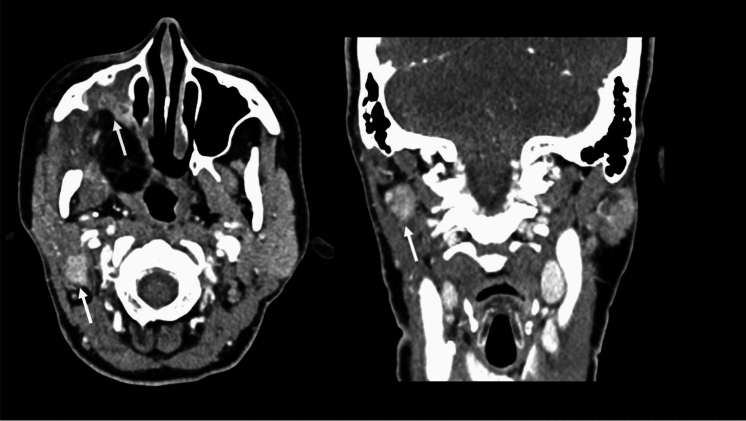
Axial and coronal views of post-operative CE-CT after 8 weeks in Patient with pT4aN3b superior right retromolar trigone SCC surgically treated with maxillectomy, neck dissection and antero-lateral thigh flap. NI-RADS 2b for *T* because there is focal ill-defined enhancement along the supero-anterior margin of the flap (yellow arrow). NI-RADS 3 for N because there is a new enlarged right retroparotid lymph node with intense enhancement and irregular margins suspicious for initial extracapsular spread (with arrow)
